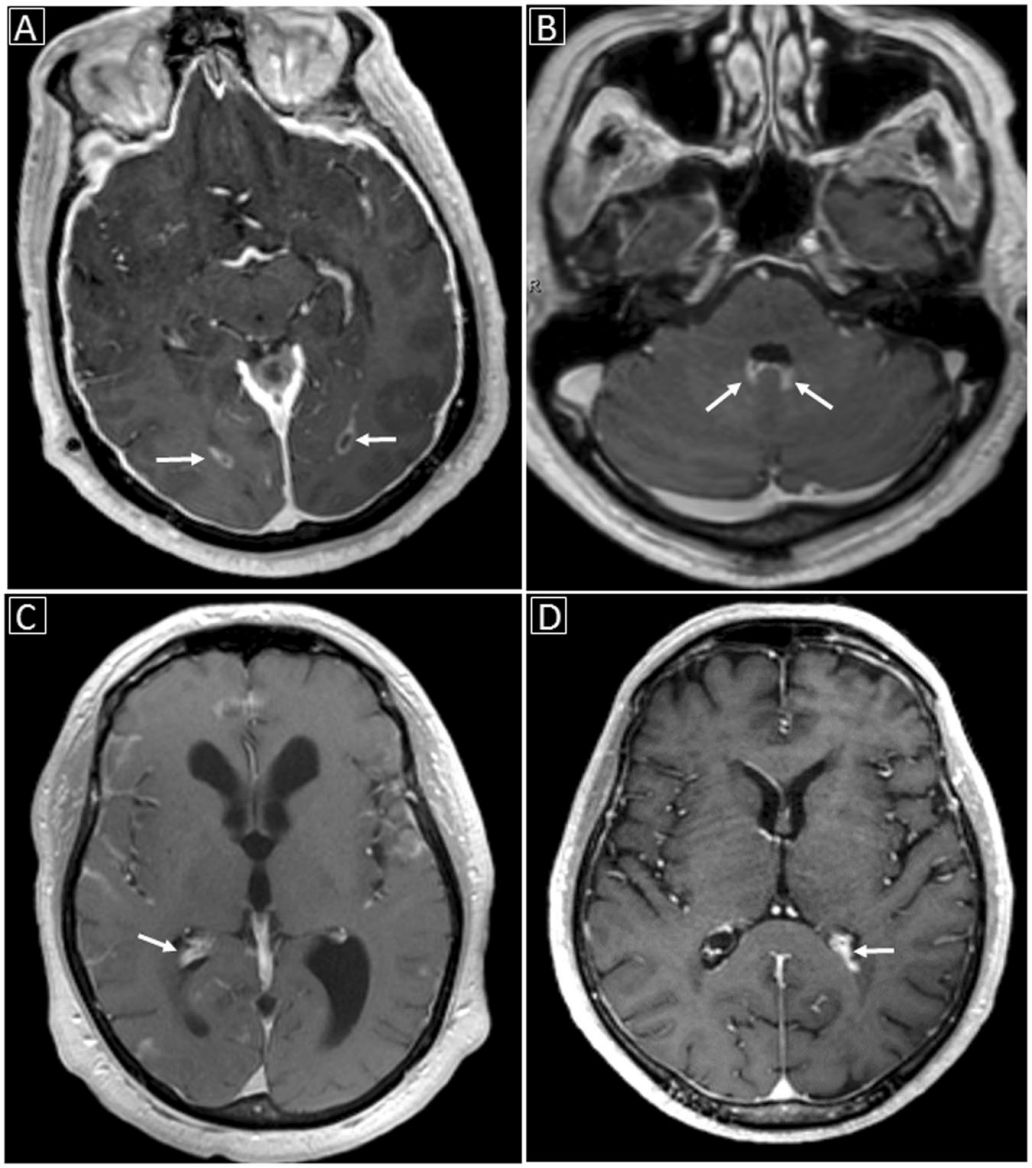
Examples of ventricular involvement in CNS cryptococcosis: (A) Abnormal ependymal enhancement along the occipital horns of the lateral ventricles bilaterally (white arrows) on enhanced T1-weighted image; (B) Abnormal ependymal enhancement along the posterior aspect of the fourth ventricle with probable associated choroid plexitis (white arrows); (C) Choroid plexitis of the right lateral ventricle (white arrow) with hydrocephalus; (D) Left lateral ventricular choroid plexitis (white arrow).
